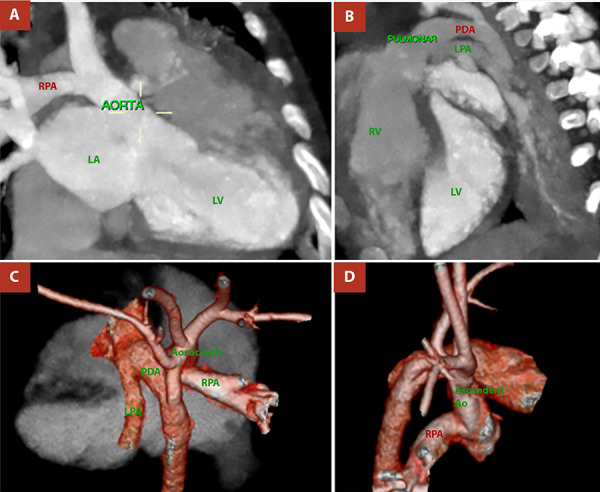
Cardiac and great-vessel computed tomographic angiography, Case 02. A) Anomalous origin of the right pulmonary artery (RPA) from the posterior wall of the ascending aorta. B) Origin of the left pulmonary artery (LPA) from the main pulmonary artery and presence of a patent ductus arteriosus (PDA). C) and D) Three-dimensional reconstruction of the heart and great vessels confirming the findings.
